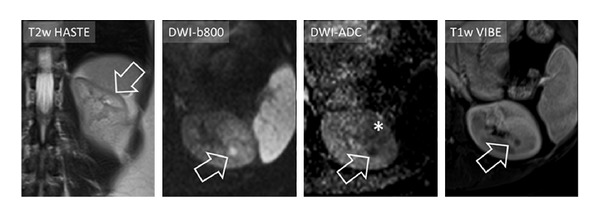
In a thirty-two-year-old female patient DWI-MRI was able to depict a small focal nephritis in the left kidney (arrow), which was surrounded by a patchy area of altered signal in DWI-b800 and DWI-ADC (asterisk in DWI-ADC). By using only T2w and T1w imaging the focal area of nephritis could easily be misinterpreted as, for example, a simple cyst.
